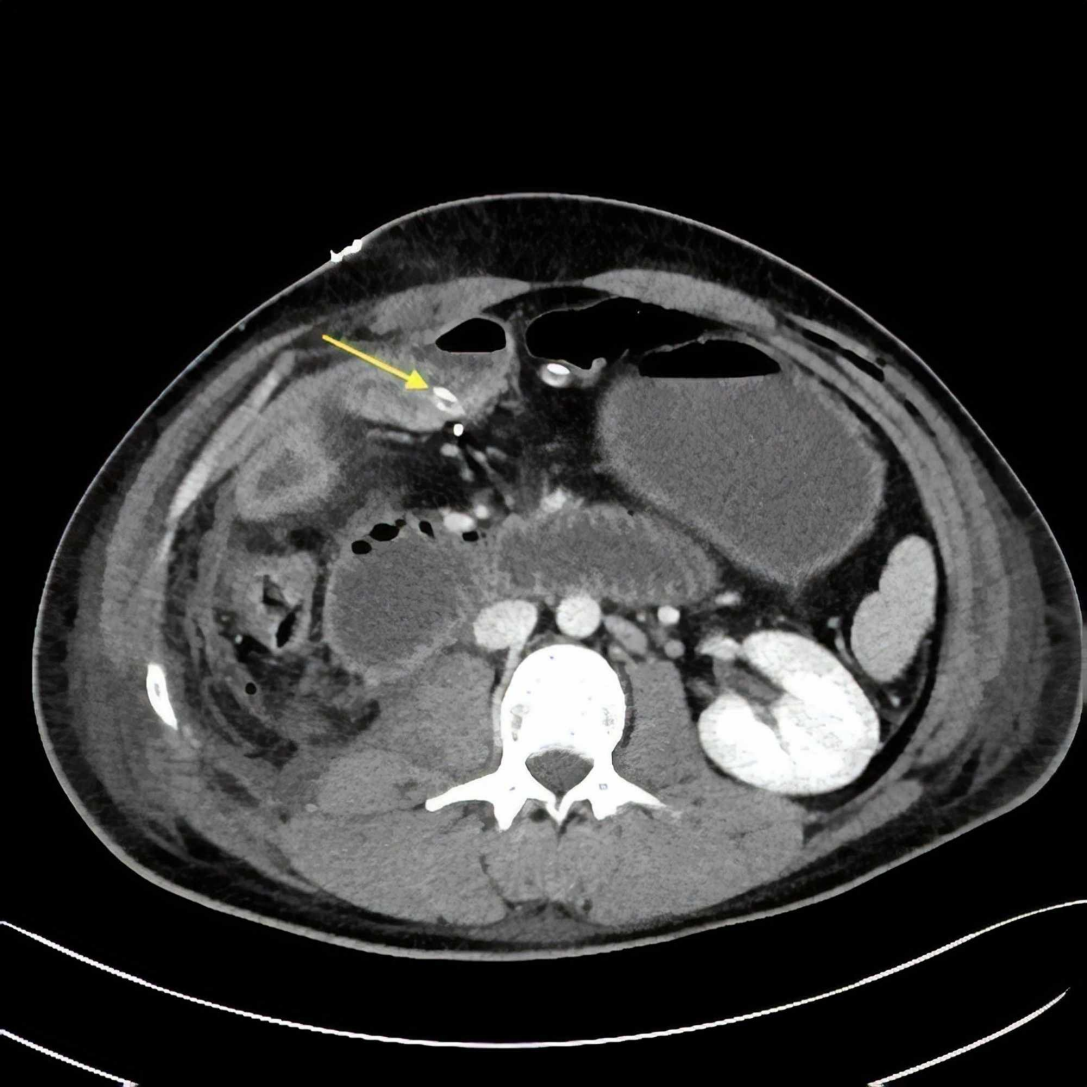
Axial view of the CT scan depicting the transition point of the obstruction close to the right abdominal Jackson-Pratt (JP) (arrow).
